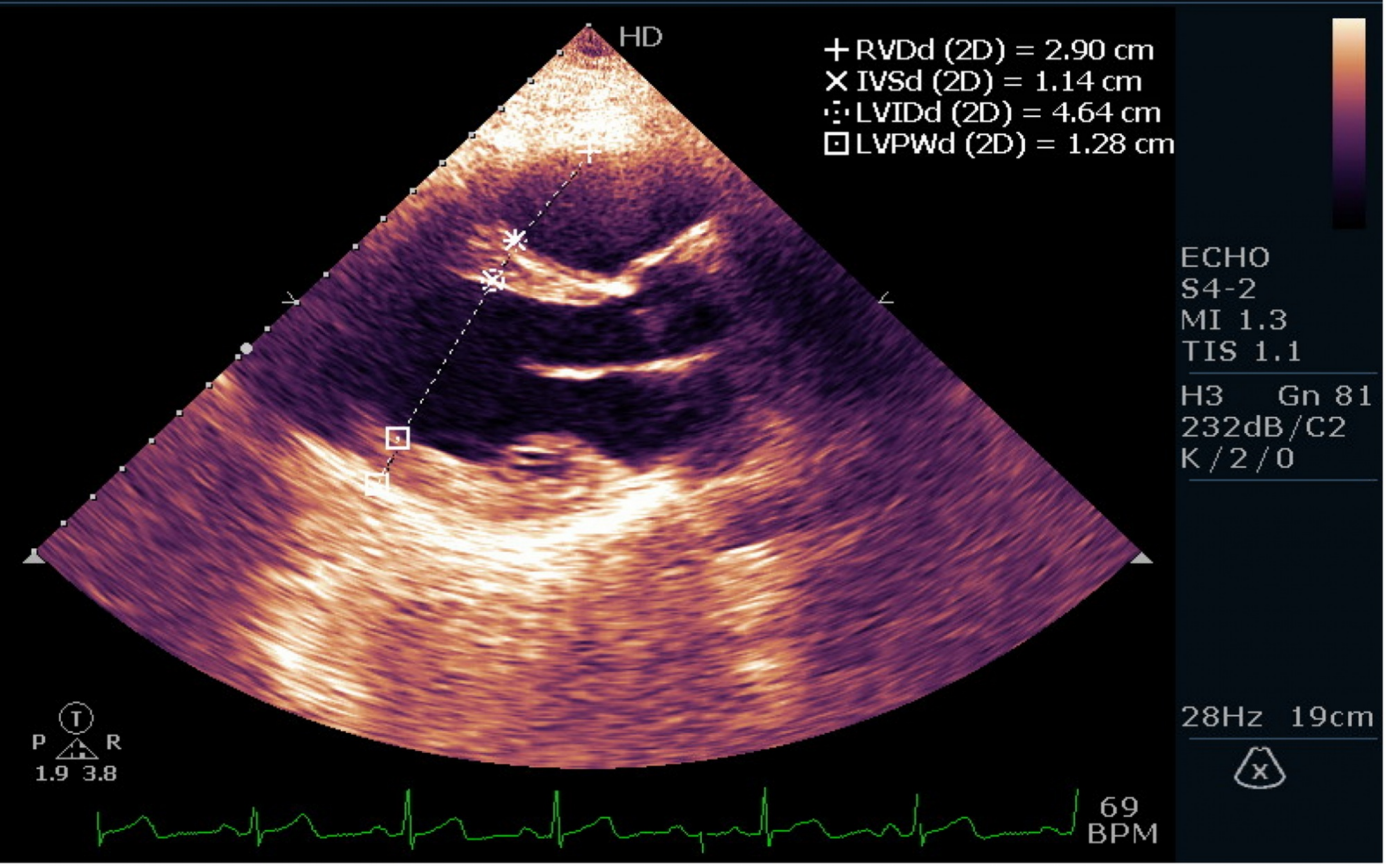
Echocardiogram for case 2.
